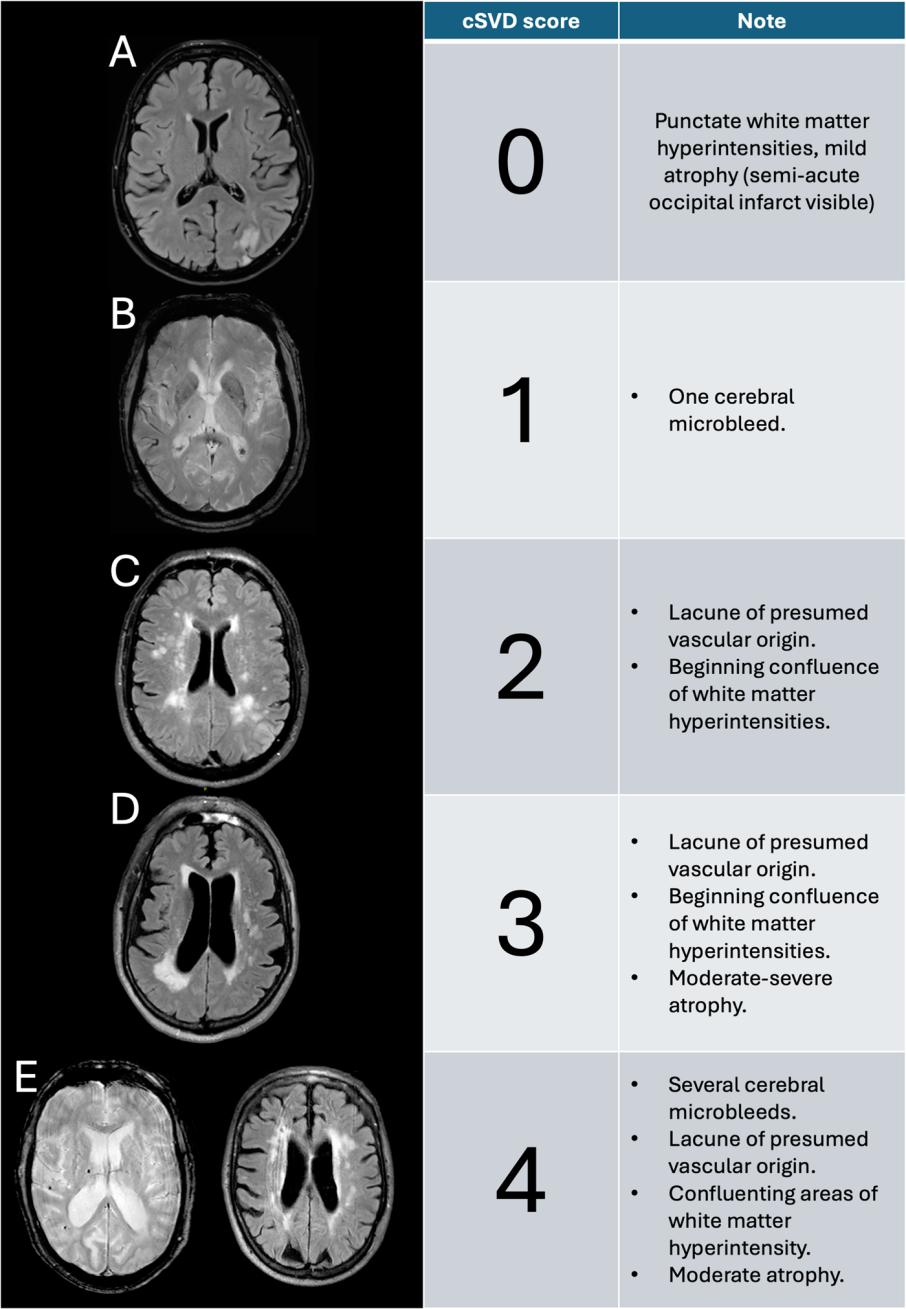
Example scores of the simplified cSVD score used from different subjects. Panel B and E (left) are from T2*‐weighted sequences, the other panels are all FLAIR sequences.
